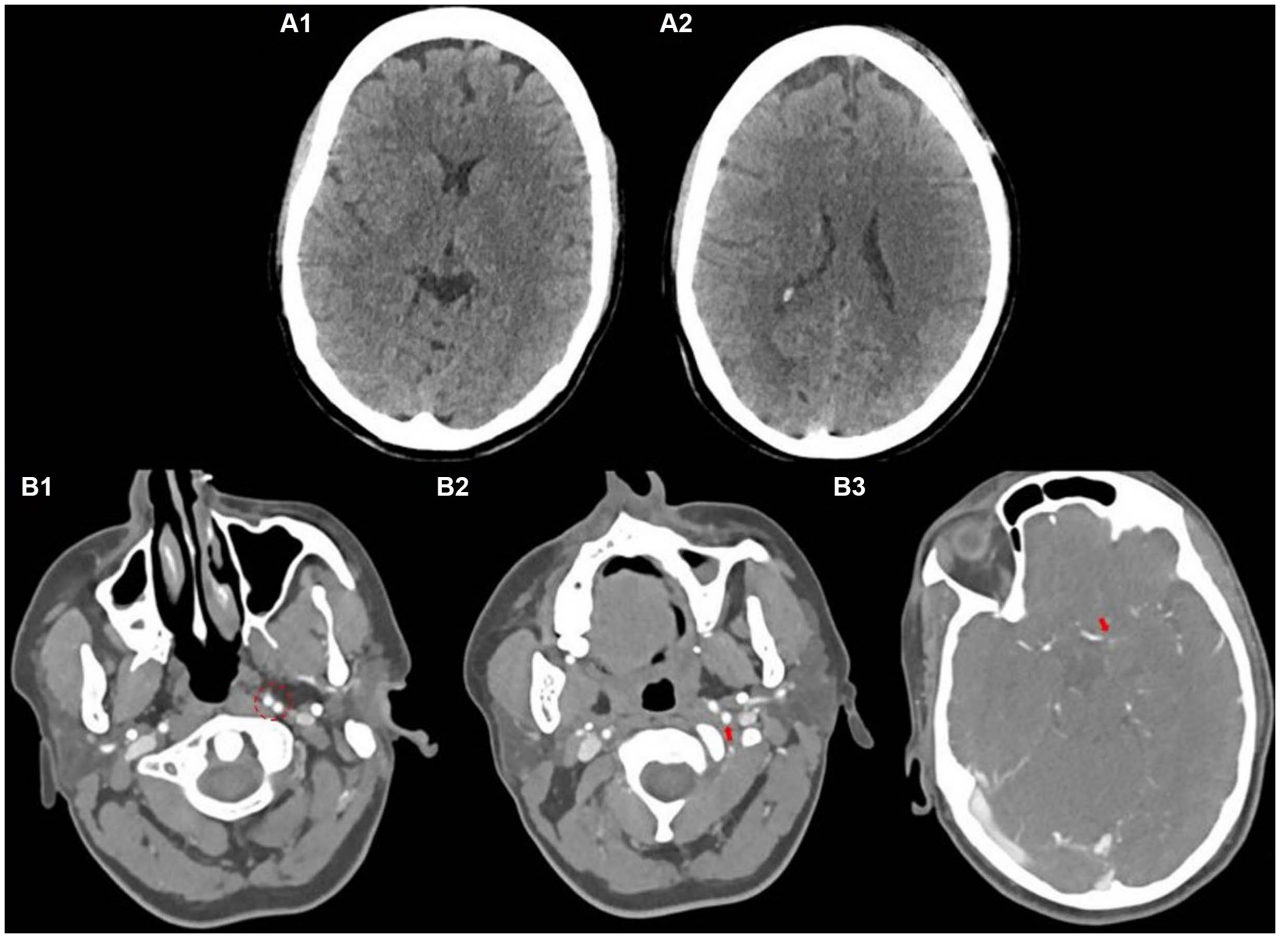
Baseline non-contrast CT scan (A), CT angiography showing bifurcating ICA (B1), persistent primitive hypoglossal artery (B2), and occluded left MCA (B3).
